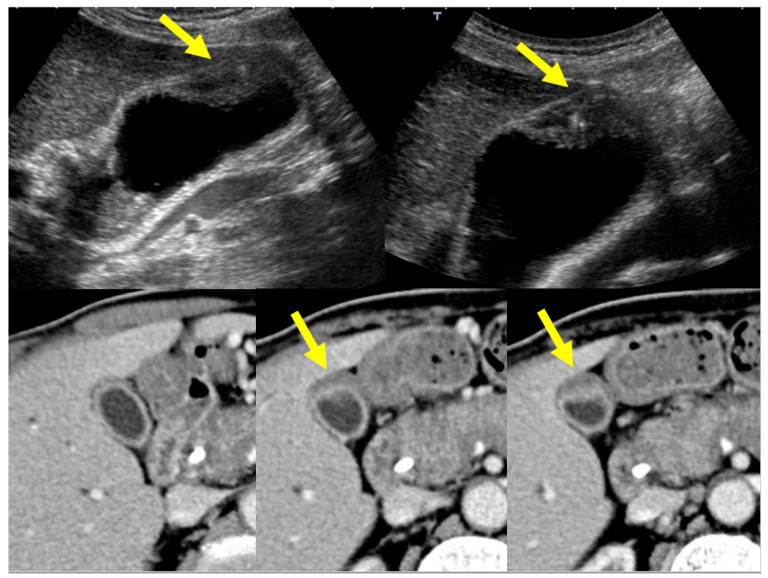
US (raised-floor type) and CT (raised-floor’ type) findings of a case with a localized type of IgG4-sclerosing cholecystitis (arrows).
